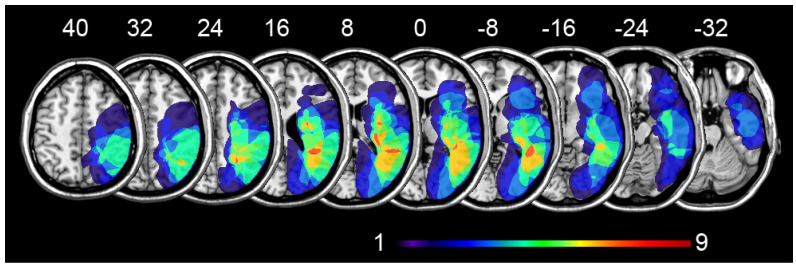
Lesion overlap plots for 12 out of 13 USN patients. Lesions were firstly drawn from CT scans in MRIcron software [45] and subsequently normalized using SPM with clinical toolbox [46]. Brain areas were labeled using the AAL template [47]. MRI axial slices were displayed according to the neurological convention. For each slice, the frequency of lesioned areas is represented through an NIH color scale ranging from 1 to 9 and superimposed on an MNI representative brain template using MRIcron. The procedure of lesion mapping is identical to those already published [48].
